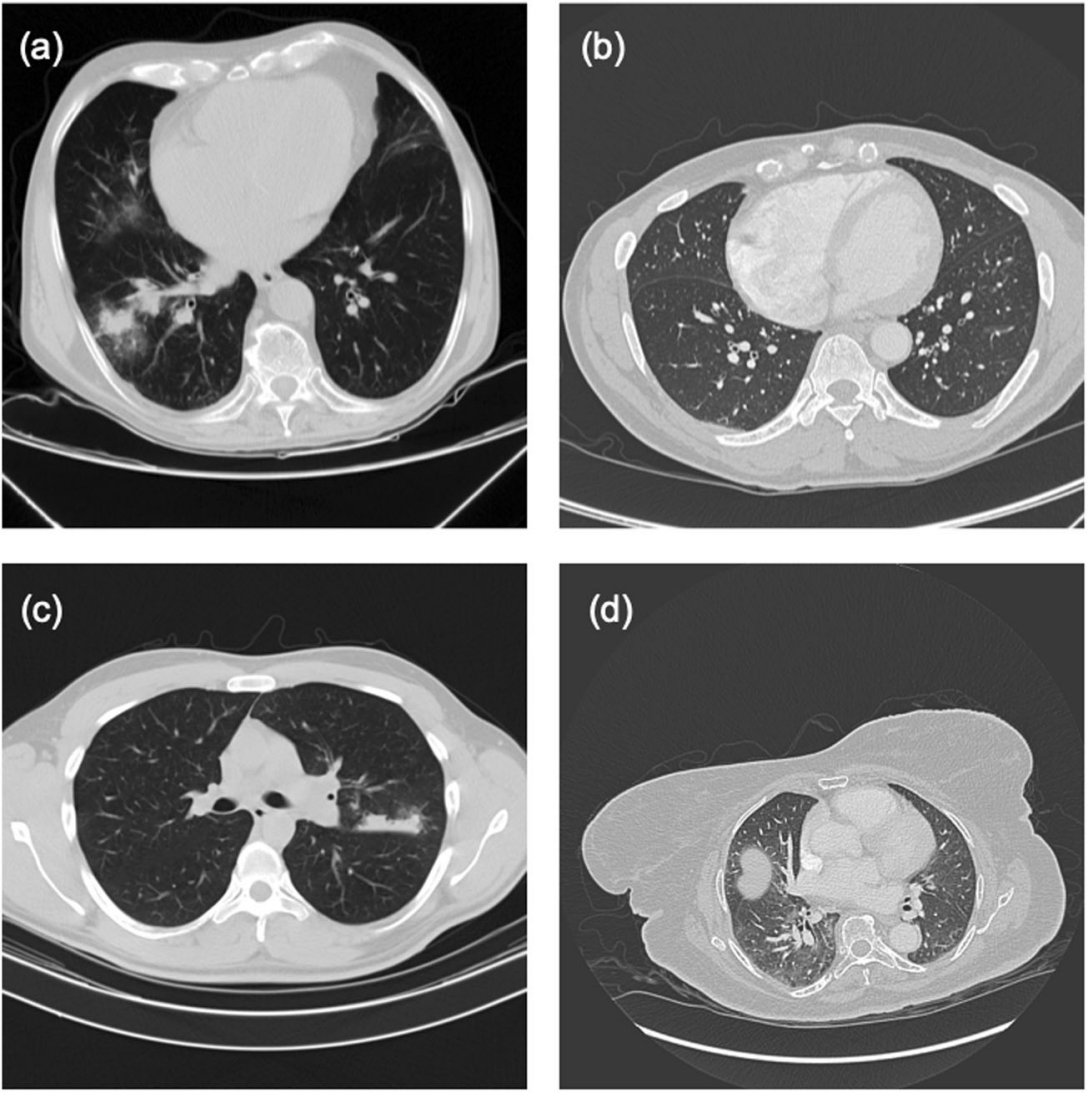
Representative examples of CT images used to test the performance of CovidCTNet versus radiologists. a A CT image of CAP. This image is misidentified as Covid-19 or control by two out of four radiologists and correctly diagnosed by CovidCTNet. b A CT image of control, that was misdiagnosed by three out of four radiologists as Covid-19 or CAP and correctly diagnosed by CovidCTNet as control. c A sample of Covid-19 that was detected as Control by CovidCTNet and as Control or CAP by three out of the entire panel of radiologists (four members). d Image of control that was misdiagnosed by the CovidCTNet as CAP and by two radiologists as Covid-19 or control. Note that, in this figure one single slide of the entire scan is shown as a representative of all CT images of a patient.
